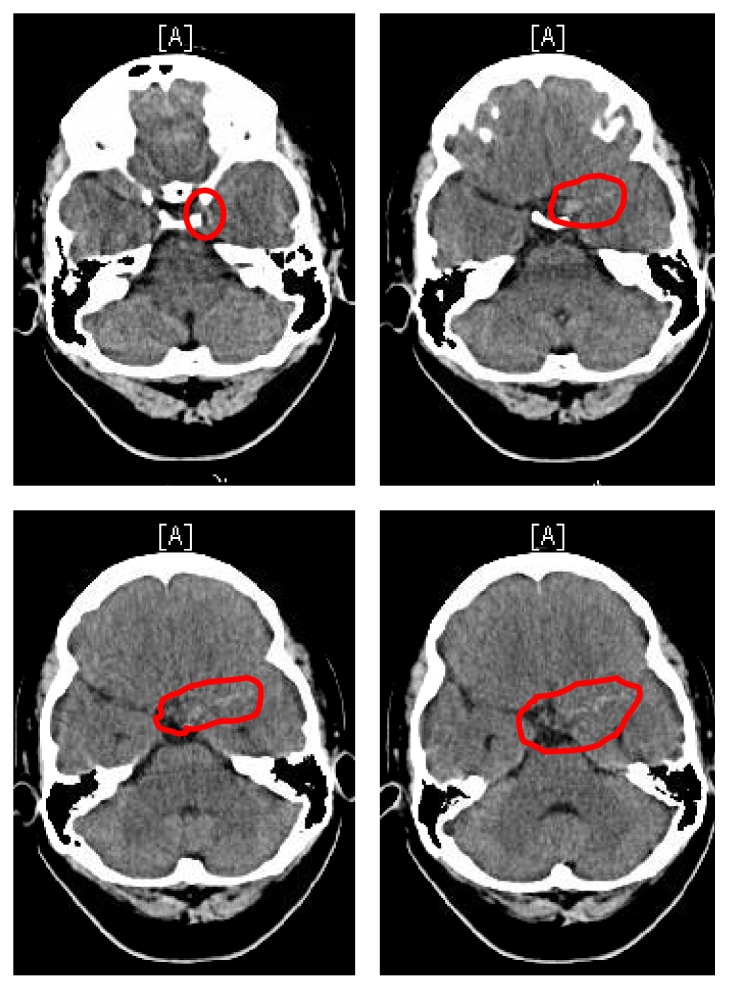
CT scan of brain without contrast axial view, on October 19, 2016, 15:51 PDT. There is mild density in the left horizontal fissure, as well as Sylvian fissure, consistent with a mild subarachnoid hemorrhage (red circles). No large acute infarction or other parenchymal lesions are identified.
